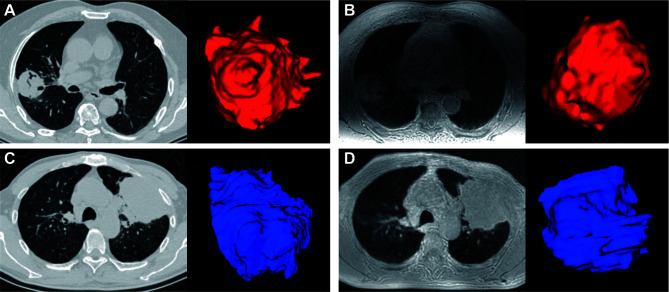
Manual segmentation of pulmonary lesions (red or blue) using volume rendering. CT (A) and ZTE-MRI (B) in the axial view showed that the measured volumes of a lung tumor in the right upper lobe were 40.7 and 46.6 mm^3^, respectively; CT (C) and ZTE-MRI (D) in the axial view show that the measured volumes of another lung tumor in the left upper lobe were 138.8 and 145.8 mm^3^, respectively.
